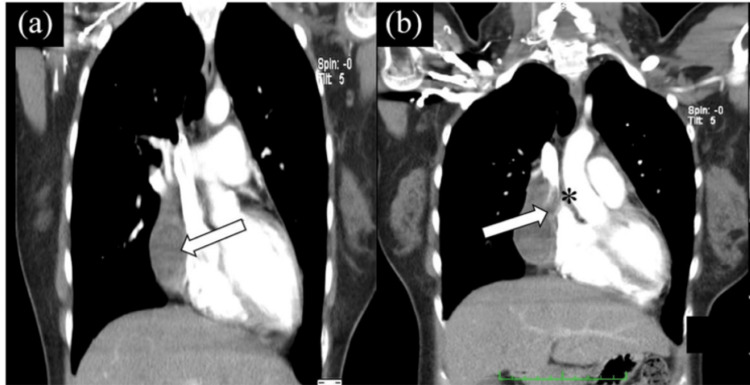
CT scan shows expanding anterior chest hematoma (arrow) compressing SVC (asterisk): a) expanding hematoma compressing SVC; b) collateral vessels visualized Source: Saboe et al. [4] SVC: superior vena cava
